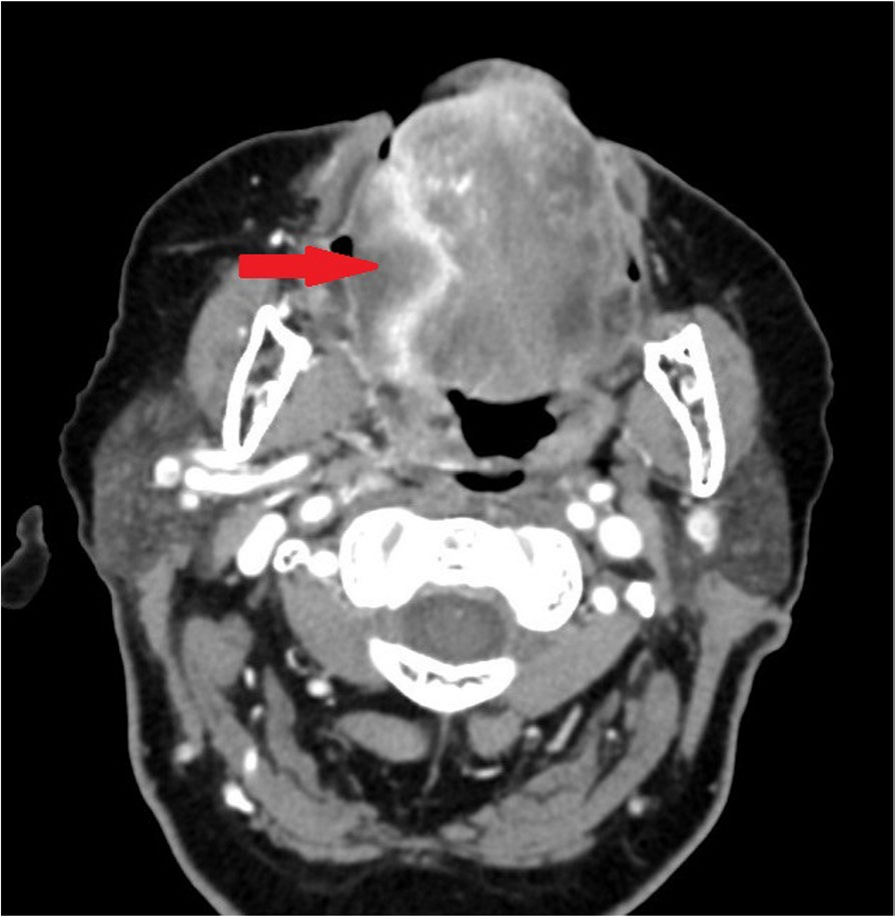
CT axial view of extensive local recurrence after radiotherapy of the tongue SCC on the right side (red arrow)
